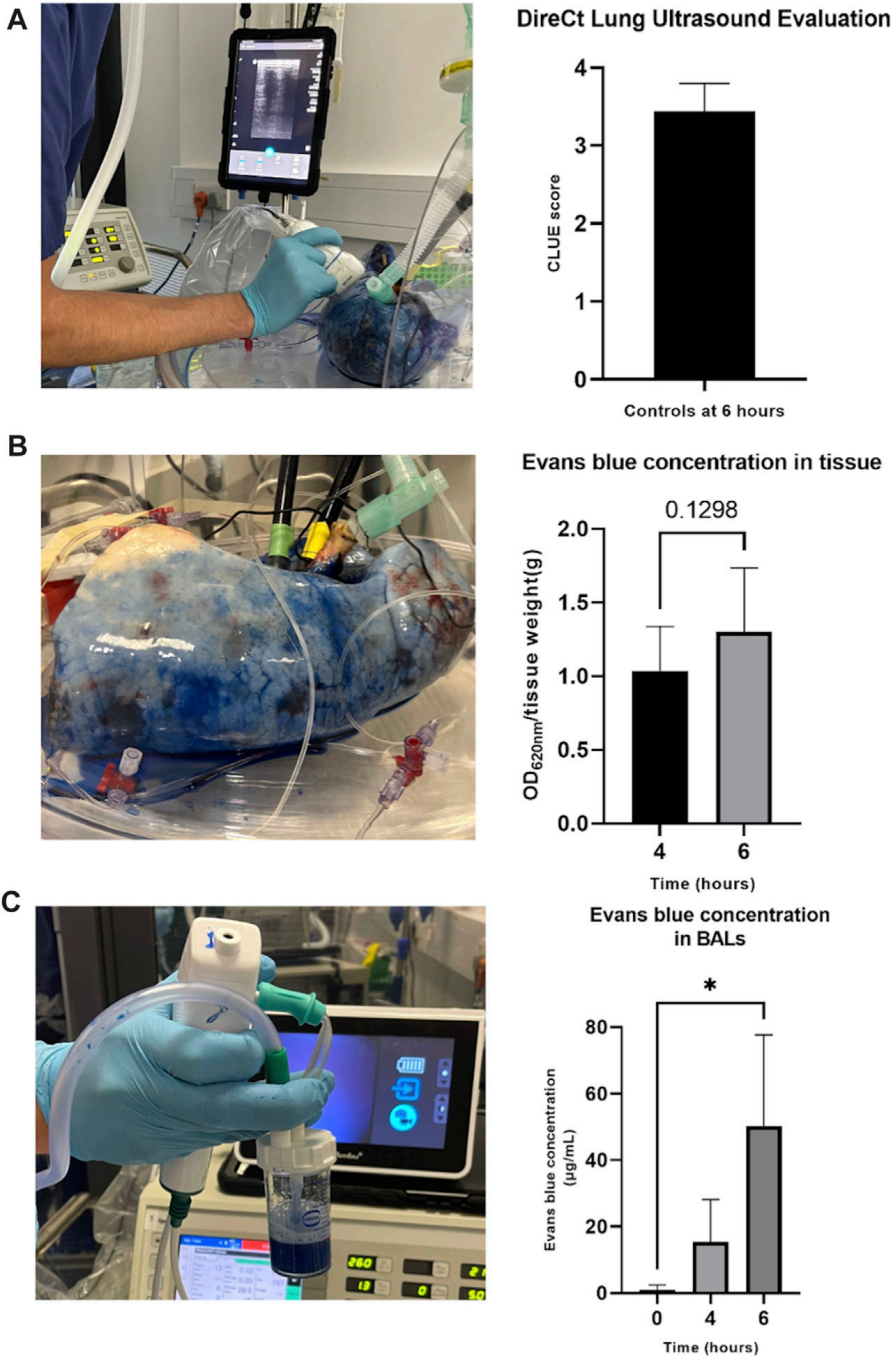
Assessment of pulmonary edema/extravascular lung water during perfusion. DireCt Lung Ultrasound Evaluation (CLUE) was performed by using ultrasound to assess 14 (left) or 16 (right) areas of the lung, providing an average CLUE score (A). Evans blue concentration in tissue was analyzed by placing biopsy samples in formamide and heating at 55°C for 24 h before centrifuging, transferring supernatant samples to a 96-well plate, and reading using a spectrophotometer at 620 nm (B). Evans blue concentration was measured in BAL samples by centrifugation, placing the supernatant in a 96-well plate and reading alongside a standard curve on the spectrophotometer at 620 nm (C). The data are expressed as mean ± SD, and analyzed by a Student’s paired t-test (B) or one-way repeated measures ANOVA and Dunnett’s multiple comparison test (C). The data presented are *n* = 6 unpaired control lungs from six independent split-lung perfusions. p* < 0.05.
